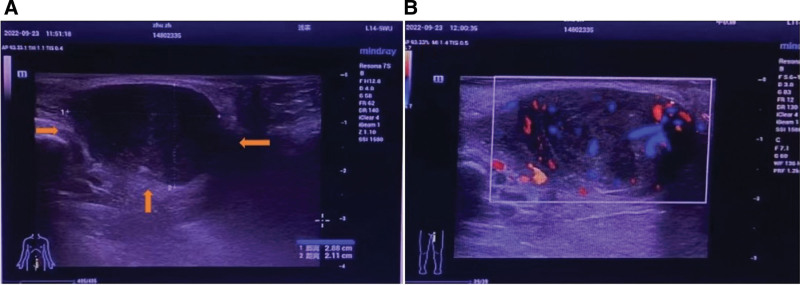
Ultrasound showed a solid mass in the posterior vaginal wall measuring 2.88 × 2.11 cm (A), Color Doppler ultrasound image showing the border (yellow arrows), and vascular nature of vulvar leiomyoma (B).
